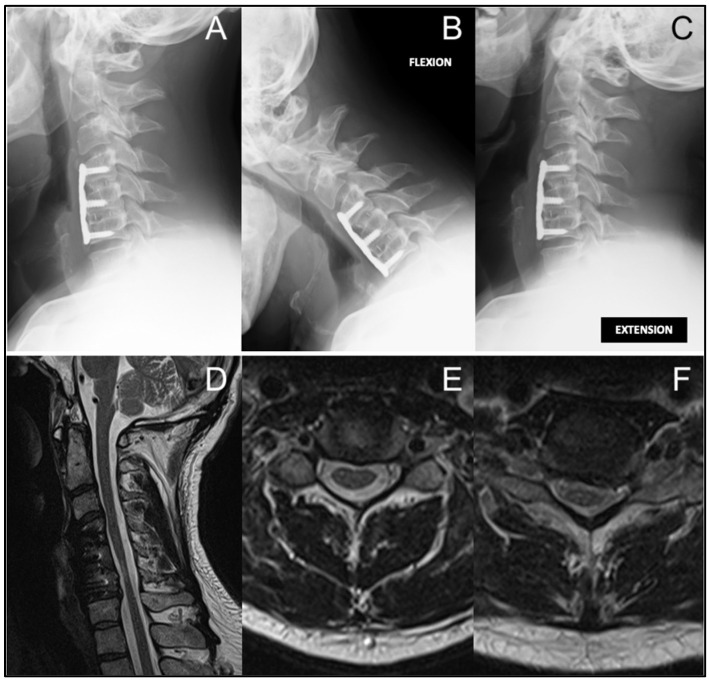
(A–C) One-year follow-up lateral cervical radiographs ((A) neutral, (B) flexion, (C) extension) showing intact anterior construct without displacement or segmental instability at C3–C4. (D–F) One-year follow-up cervical T2-weighted MRI ((D) sagittal, (E,F) axial) showing intramedullary T2 hyperintensity at C3–C4 (E), and a new left C6–C7 foraminal disc herniation compressing the C7 nerve root (F).
